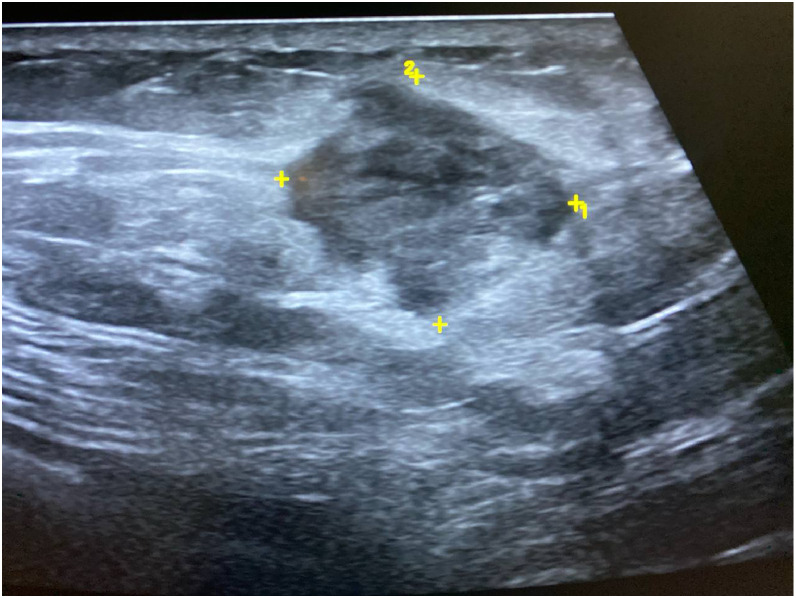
Ultrasound of the left axilla showing an oval, heterogeneously hypoechoic mass oriented parallel to the skin and lying 3-4 mm deep to it. The margins are macrolobulated with an angular upper margin, and no posterior acoustic features are seen. A central echogenic line is present within the lesion. Electronic calipers mark the lesion dimensions.
